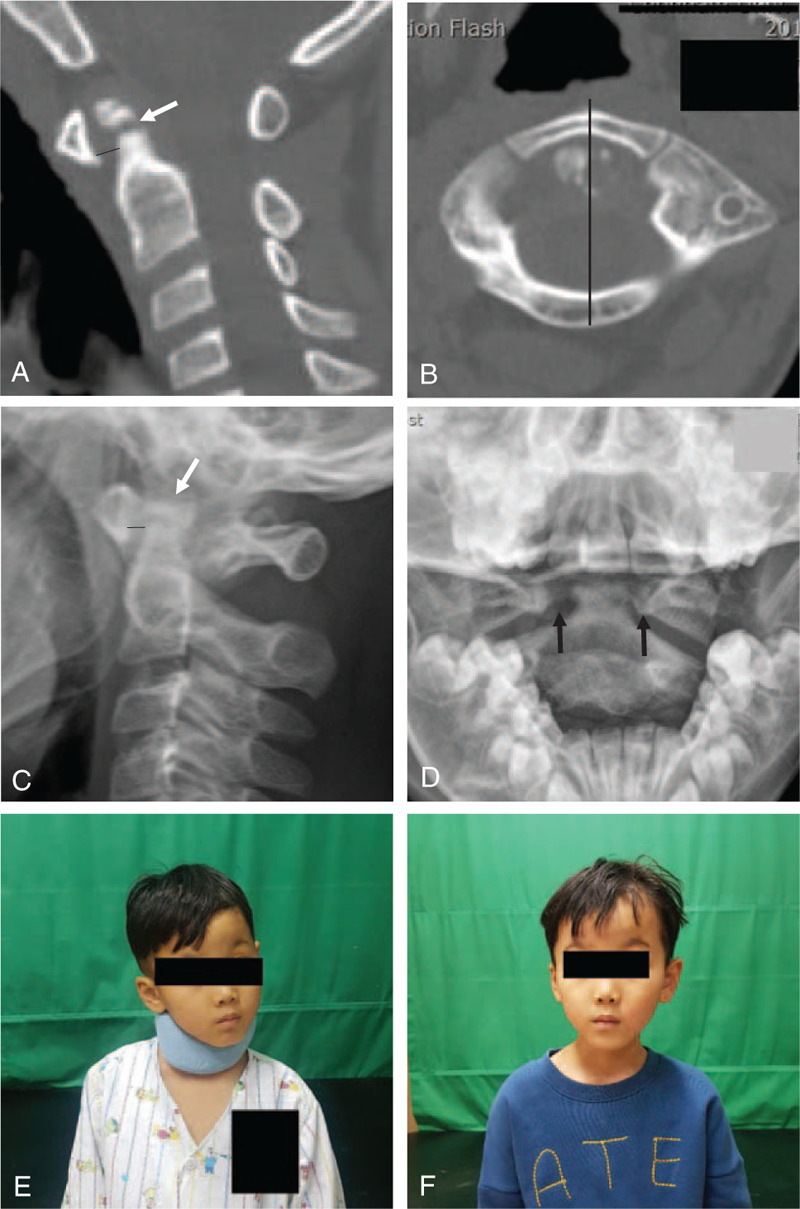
One month after closed reduction, follow-up sagittal reconstructed and axial (A and B) computed tomography (CT) scans clearly showed successful reduction of transverse dens fracture, decreased ADI of 3 mm (dark line), and correction of type II atlanto-axial rotatory subluxation (AARS). Seven months after closed reduction, follow-up lateral radiograph and open mouth view (C and D) showed a well maintained reduced transverse dens fracture with ossiculum terminale (white arrow), corrected type II AARS (dark arrows), and normalization of atlanto-dental interval (ADI) of 2 mm (dark line). However, transverse dens fracture was not still united. Compared to initial traumatic torticollis due to type II AARS (E), a follow-up clinical photo (F) showed normal neck posture with correction of torticollis.
